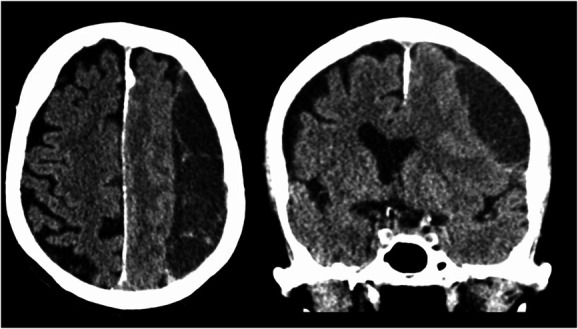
Computed tomography scan of a left-sided chronic subdural hematoma in an axial and coronal plane.
